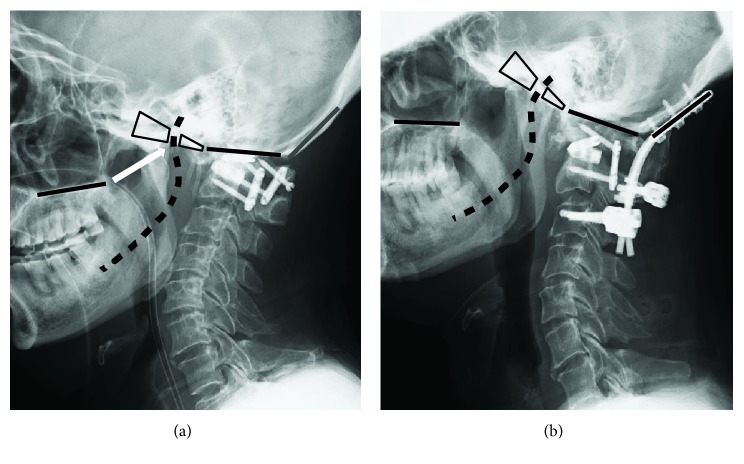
(a) Pre- and (b) postoperative lateral cervical X-rays showing the surgical plan for the change of direction of hypoglossal nerve (black dotted lines). The white arrow points the place at which the nerve exits from the hypoglossal foramen and is stretched backward due to the O-C kyphosis.
